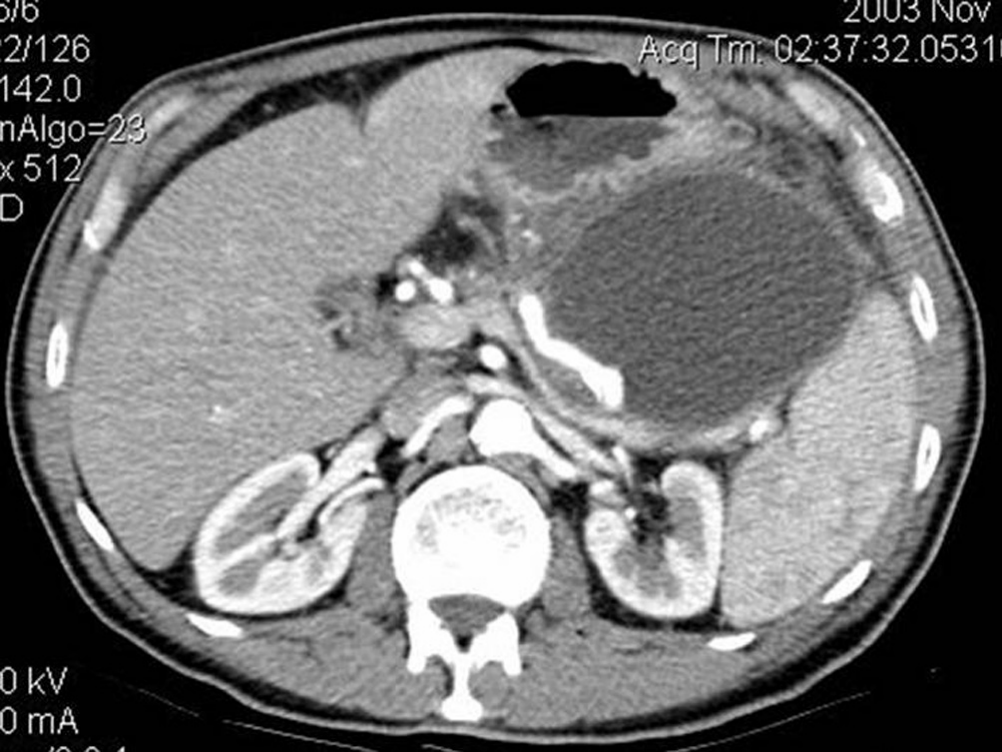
Patient at admission: arterial phase CT shows normal splenic artery immediately behind a large pancreatic pseudocyst.
